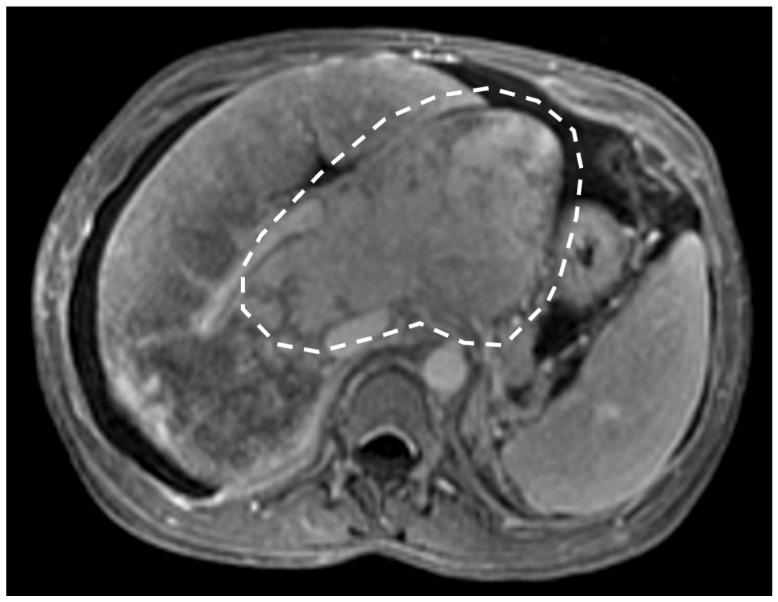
Axial portal venous phase MR image shows huge enlargement and hyperperfusion of caudate lobe (white circle). This is an important finding because sinusoidal congestion does not develop in the caudate lobe since it presents its own venous drainage.
